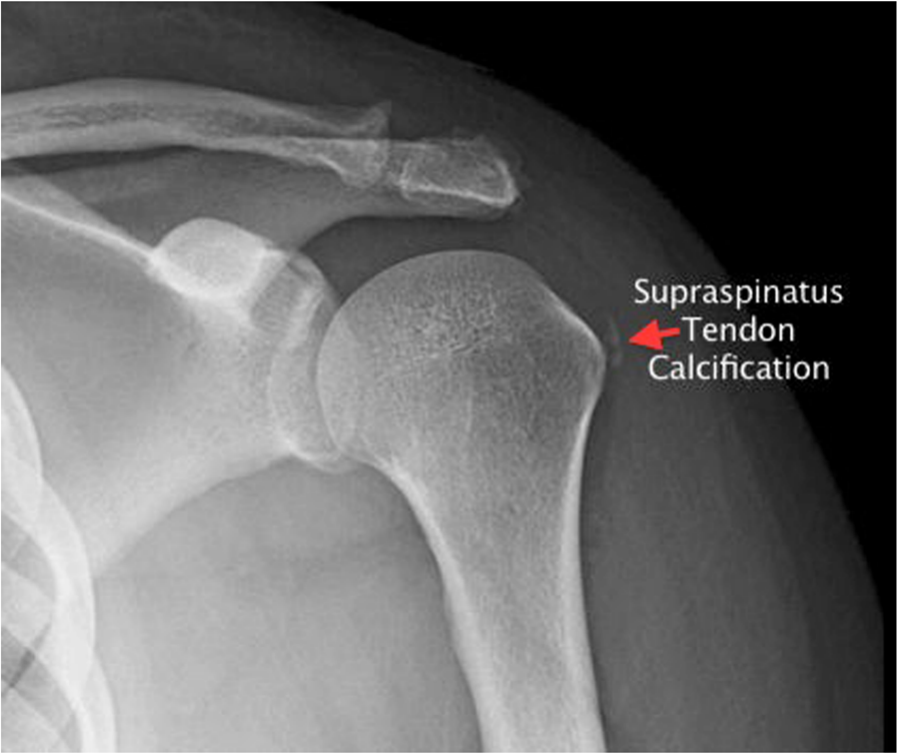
Plain film radiograph of the proximal humerus with supraspinatus tendon calcification.
